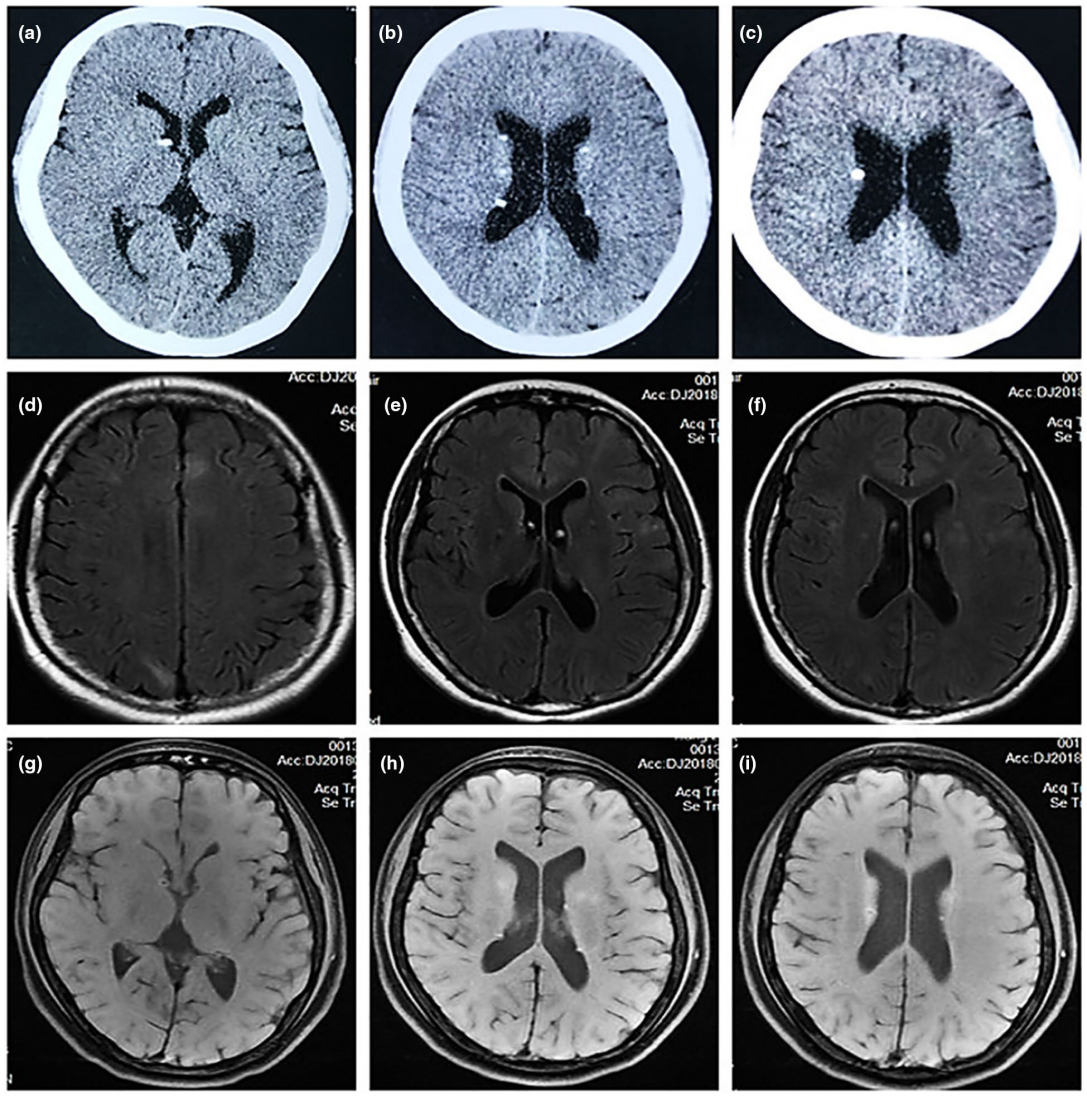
Imaging appearance of the Central Nervous System. (a–c) Axial computed tomography of the brain, demonstrating subependymal nodules. (d–f) Axial magnetic resonance imaging (MRI) (T2 flair) of the brain, demonstrating the cortical dysplasia (tubers and radial migration lines). (g–i) Axial MRI (T1 + contrast) of the brain, demonstrating the subependymal nodules
